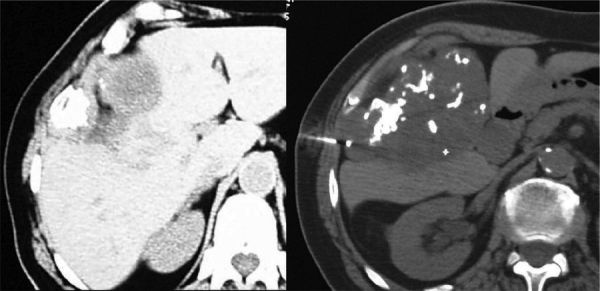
Axial CT scan reveals a mass with coarse calcifications originating from the chest wall. A CT-guided biopsy identified the mass as a chondral tumor.
